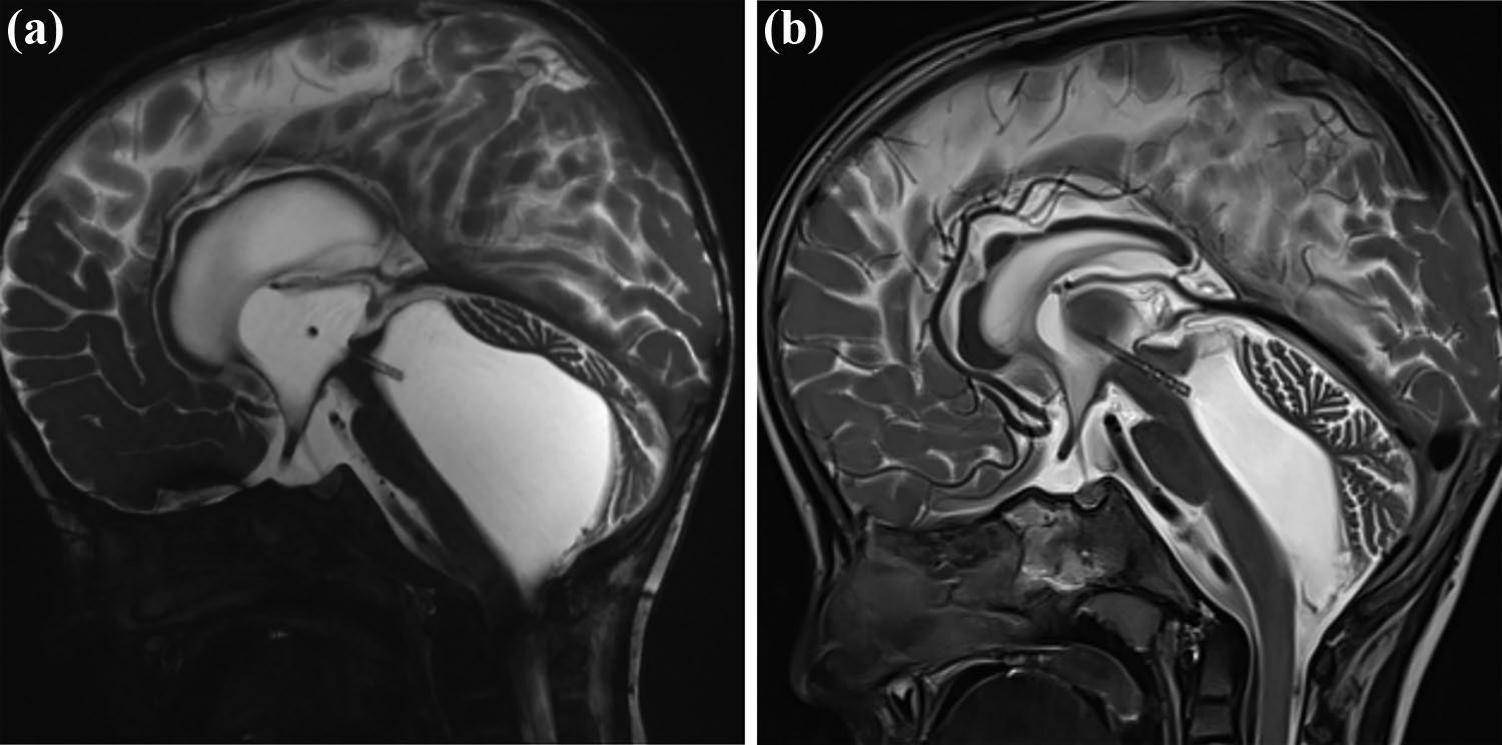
Postoperative control MR images. MR images were taken four days after surgery (a) and 14 months after surgery (b), confirming the trans-aqueductal position and functionality of the stent. The size of the fourth ventricle regressed revenant resulting in the resolving of previous brain compression. The prepontine cistern was again definable. The cerebellum was unfolded. All figures were created using Adobe Photoshop
